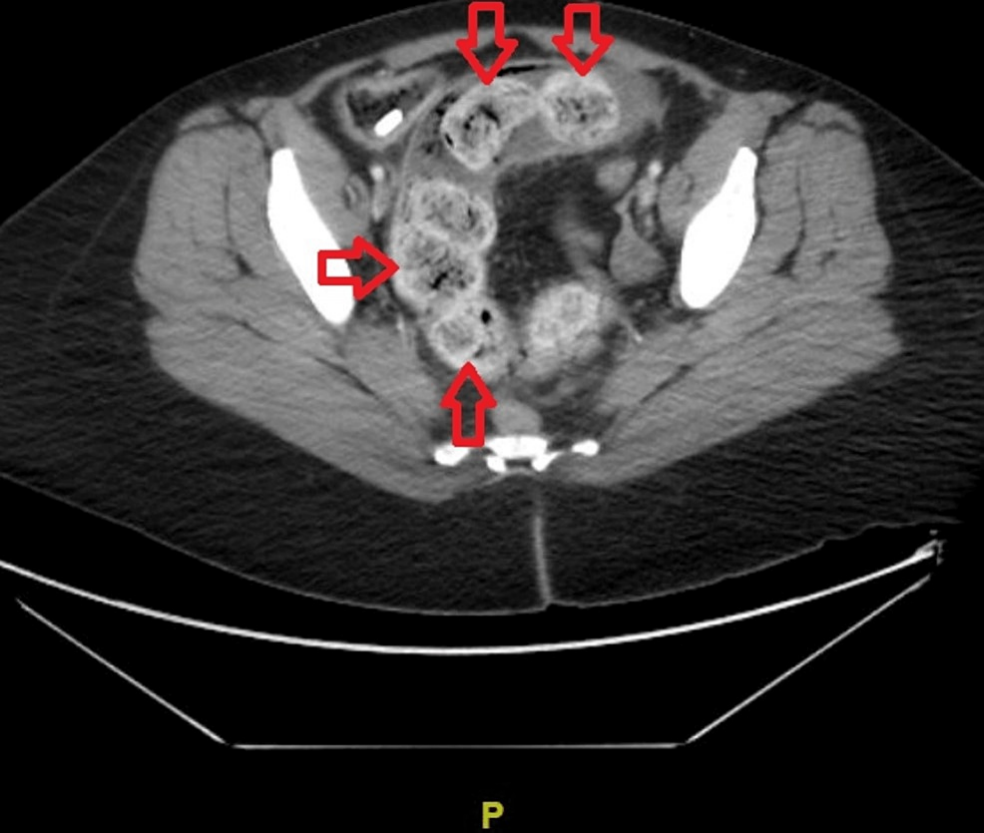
Axial view of contrast-enhanced computed tomography of the abdomen and pelvis indicating moderate to large burden of stool (Indicated by red arrows) throughout the colon
